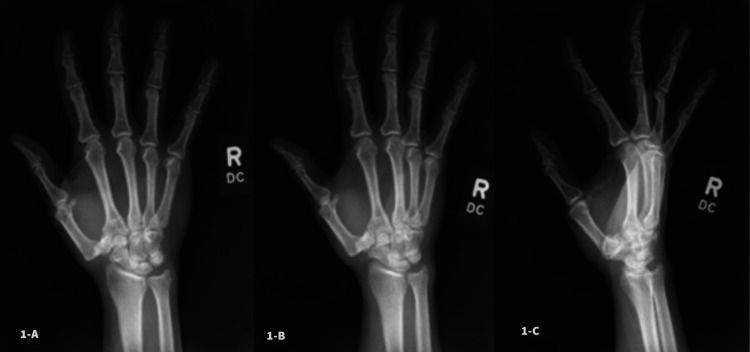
Preoperative X-rays in posteroanterior (Fig. 1-A), oblique (Fig. 1-B), and lateral (Fig. 1-C) views demonstrating dislocation of the thumb CMC joint
